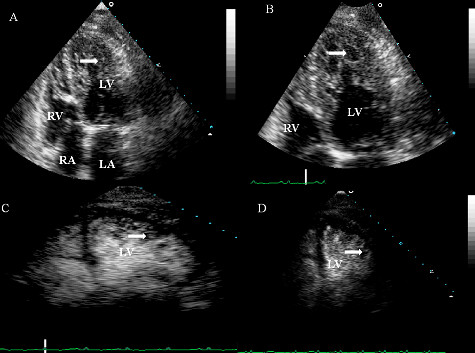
Transthoracic echocardiogram (A, B, C, D) four chamber view with albumin contrast showing numerous trabeculations (white arrow) in the left ventricular apex, along with deep intertrabecular recesses. (RA- right atrium, LA- Left atrium, RV-right ventricle, LV- left ventricle).
